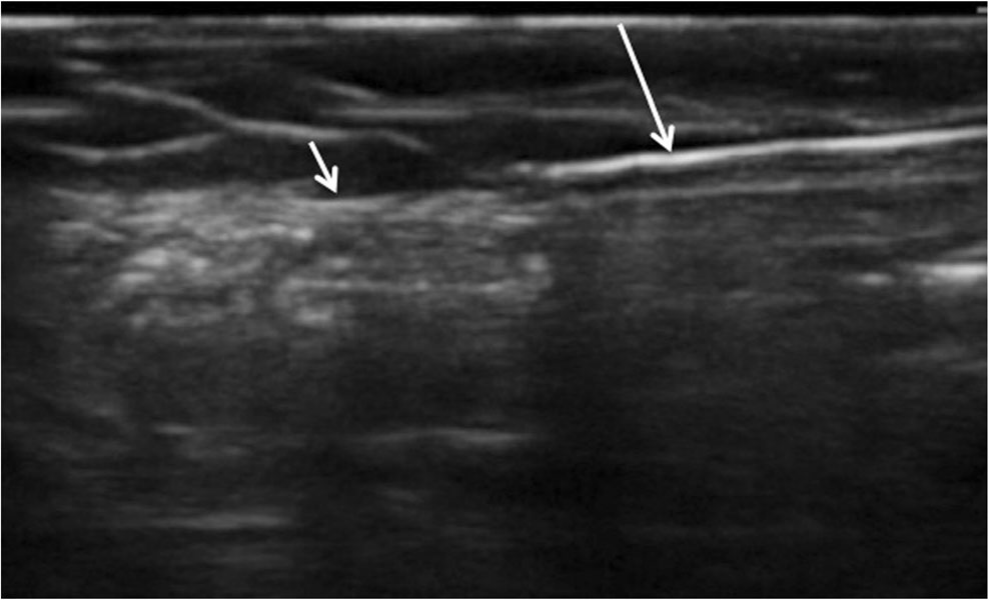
Real-time ultrasound image of blunt tunneling device (*long arrow*) passing immediately superficial to fascial plain (*short arrow*)
